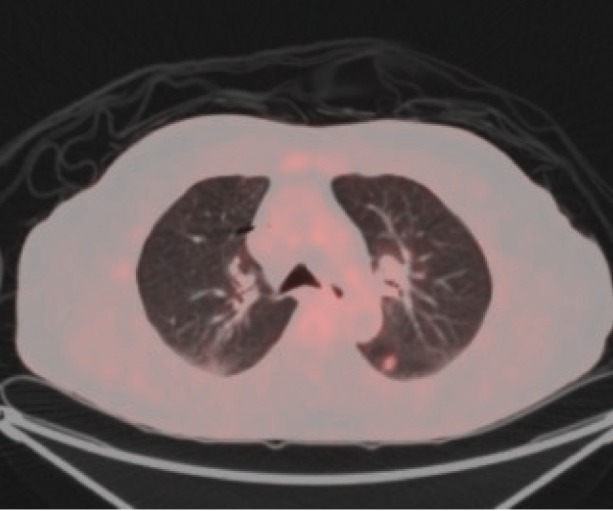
April 2013 PET-CT scan reveals increased metabolic activity in the size of the left lower-lobe lung lesion.
